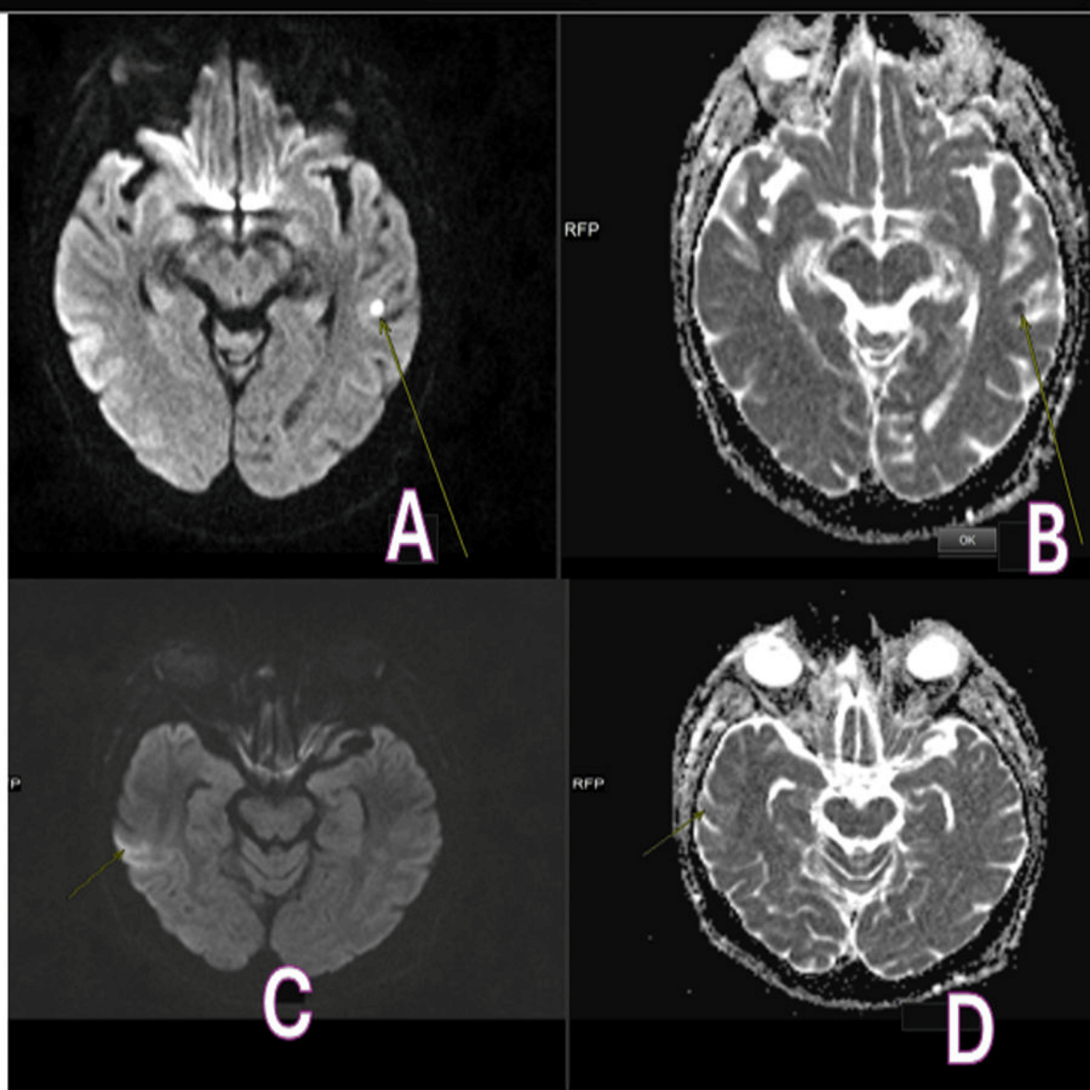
Head MRI with and without contrast. (A) A tiny focal hyperintensity (arrow) is seen on T1-weighted images, showing a blooming artefact on the gradient sequence in the left posterior capsulo-ganglionic region. (B) A tiny focal area of filling defect (arrow) is seen in the left posterior capsulo-ganglionic region. (C) A tiny focus of restricted diffusion (arrow) is seen involving the right temporal lobe, consistent with an acute lacunar infarct. (D) A tiny hyperintense focus (bright spot) (arrow) is seen involving the right temporal lobe, consistent with an acute lacunar infarct.
